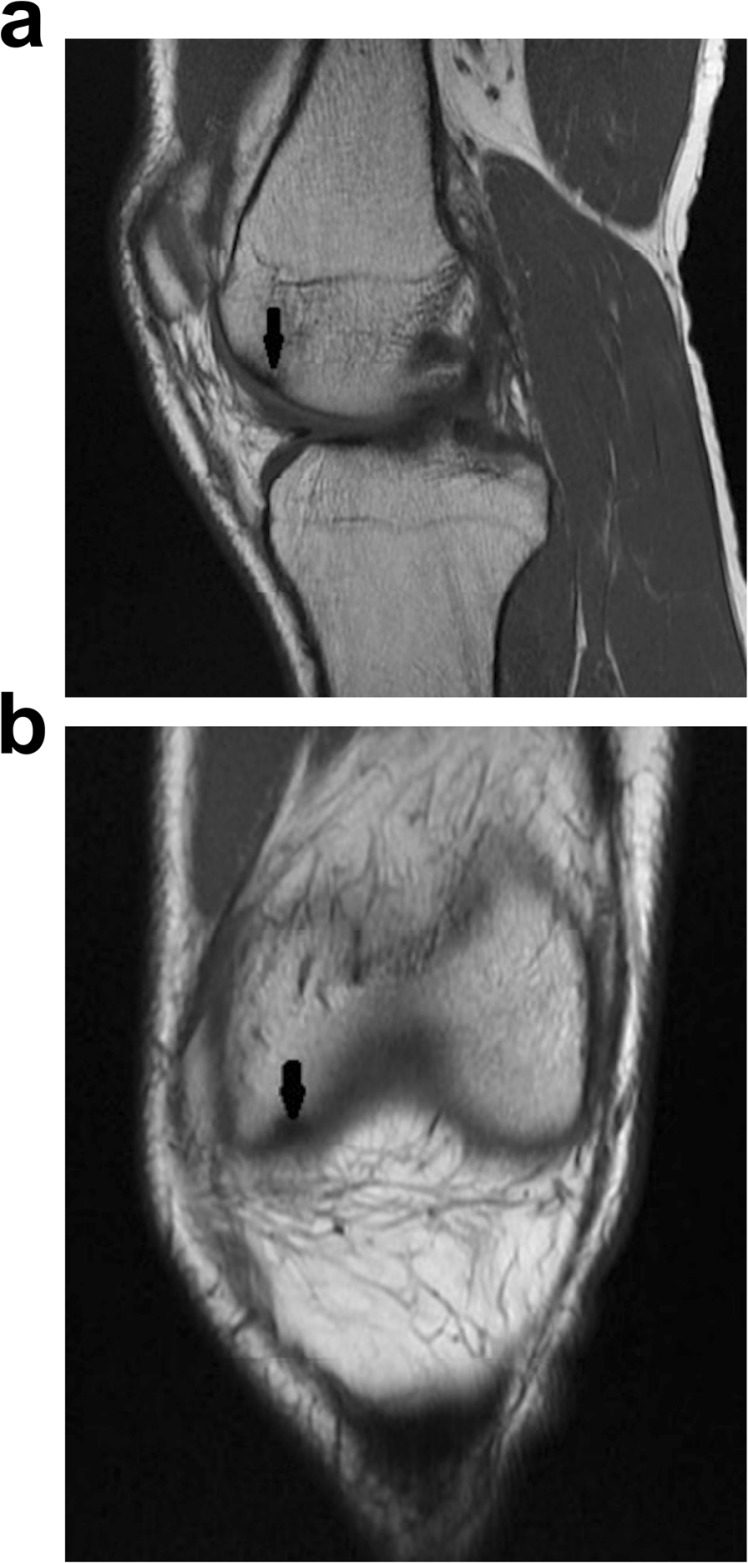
Left Knee T1 MRI showing subtle abnormality of subchondral bone in the same patient. a Sagittal view, b Coronal view
